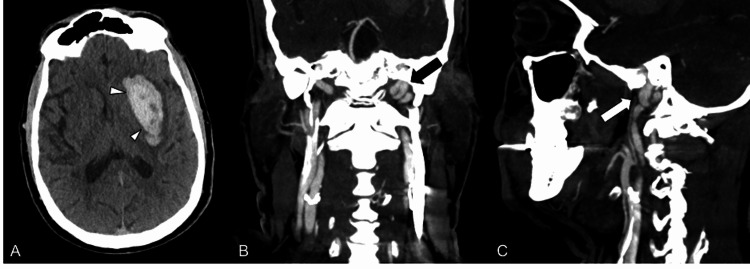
Computed Tomography Imaging Workup (A) Axial non-contrast head CT shows an intraparenchymal hematoma centered in the left basal ganglia (arrowheads) with a mild left-to-right midline shift. (B) Coronal and (C) sagittal CT angiogram images reveal a tortuous distal cervical left ICA at the skull base (black arrow in B and white arrow in C).
